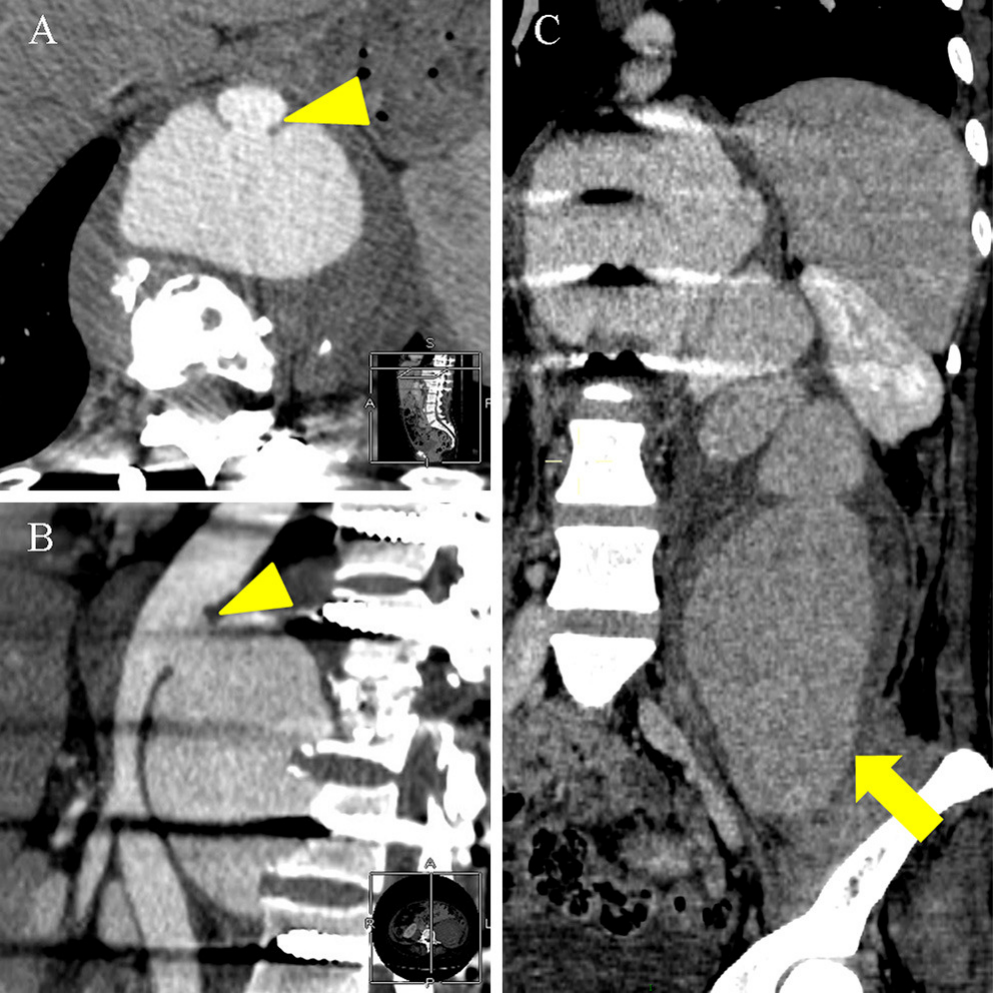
An enhanced CT scan on 1 December 2021 showed a pseudoaneurysm measuring 8.2 × 5.2 × 22.9 cm. (A,B) Axial and lateral enhanced CT scan showed the thoracic aorta communicated with the pseudoaneurysm through the rupture (arrowhead). (C) Coronal enhanced CT scan showed the pseudoaneurysm significantly enlarged into the left iliac fossa (thick arrow) compared with Enhanced MRI scan on 6 November 2021.
